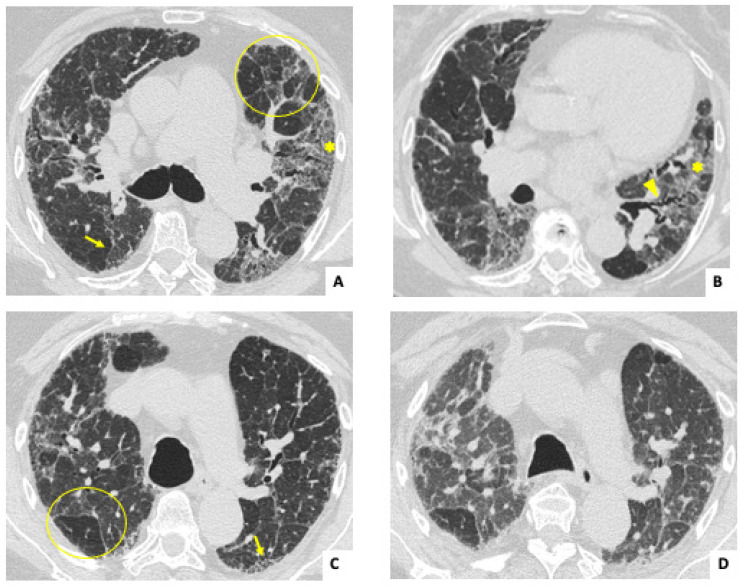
Inspiratory axial HRCT images (A–C) in a 65-year-old woman diagnosed with isolated ANCA-ILD and positive C-ANCA. Pictures show reticulation (arrows), traction bronchiectasis (arrowhead), and ground glass opacities (asterisks), with no craniocaudal gradient. Bilateral mosaic pattern (geographic regions of decreased lung density) can also be detected (circles). Expiratory CT (D) confirms the presence of air trapping, and the mosaic pattern is highlighted compared to C. Findings suggest a non-UIP fibrotic interstitial lung disease.
